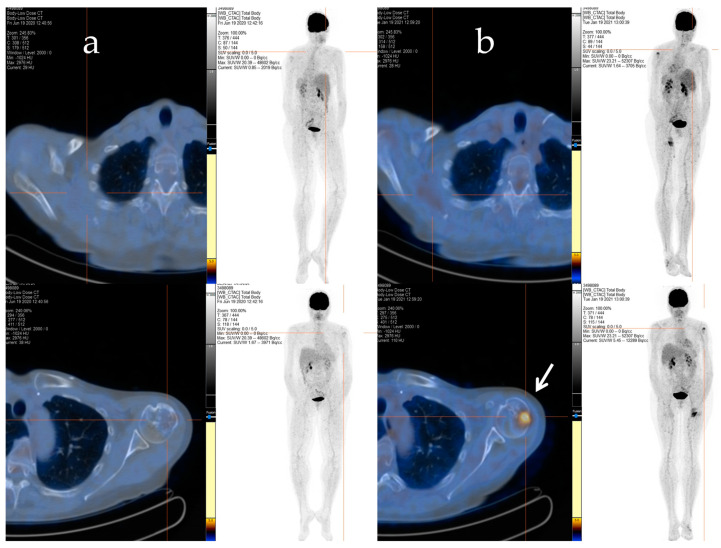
The F-18 FDG PET/CT performed after 48 cycles of trabectedin treatment (a) and after the period of trabectedin suspension (b). The main tumour lesions are indicated by white arrows.
